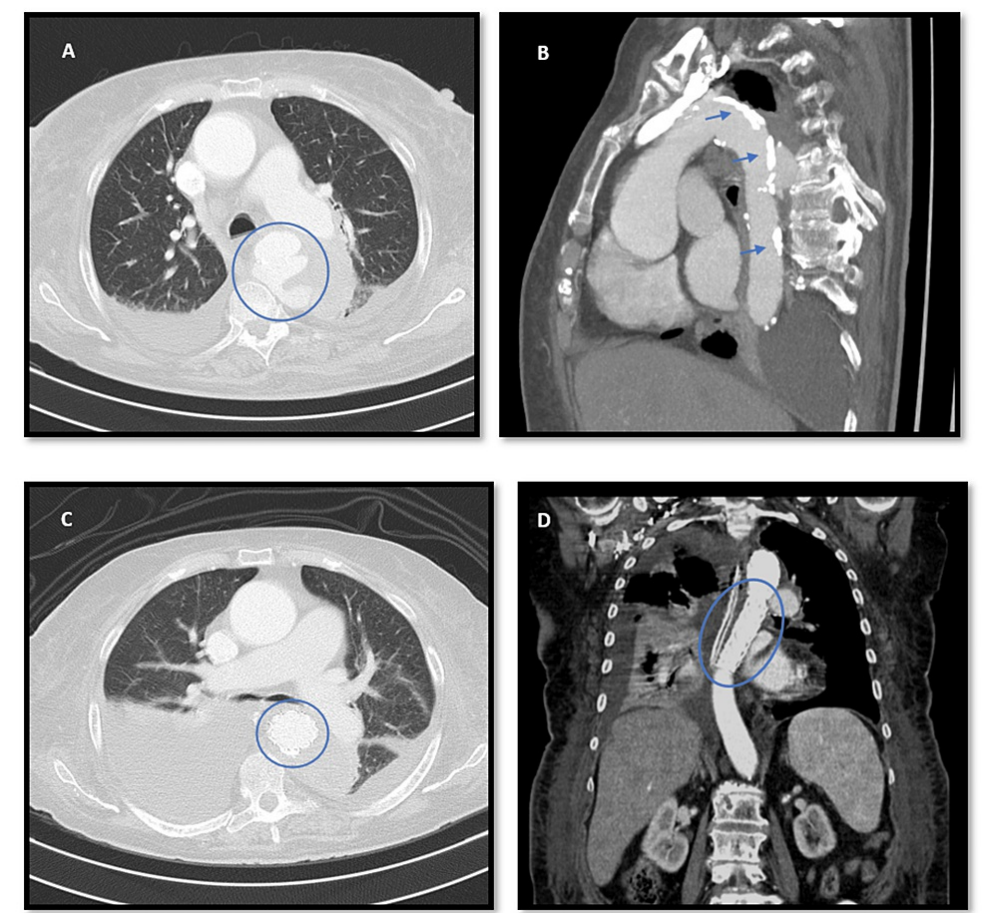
Axial (A) and sagittal (B) computed tomography images of the chest with administration of intravenous contrast agent, where it is documented the Type I Crawford aortic aneurysm. (B) Identifying the atherosclerotic plaque. (C, axial) and (D, coronal) show the repaired aneurysm.
